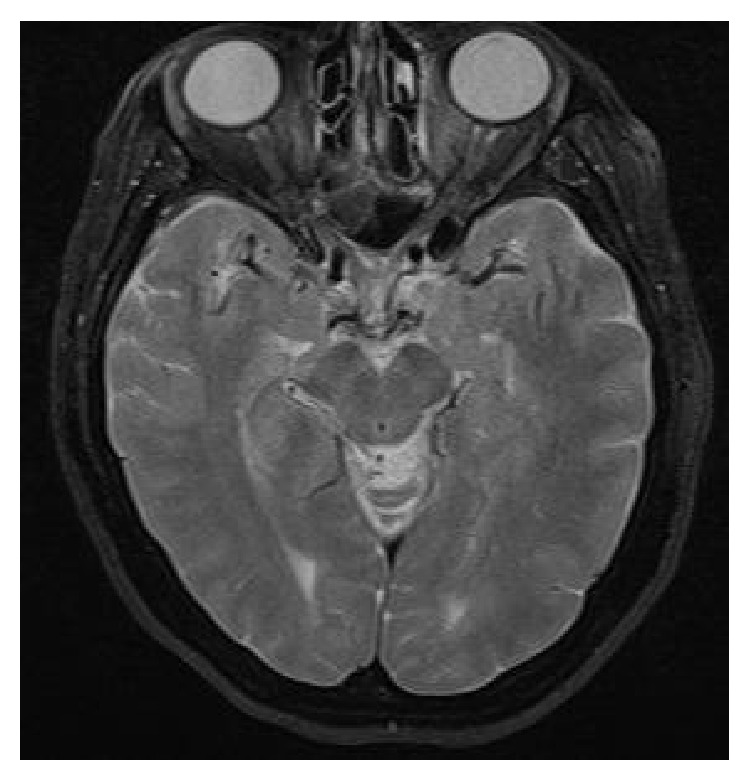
Cranial magnetic resonance imaging one month later (the neuropathy in the left optic nerve was regressed).
